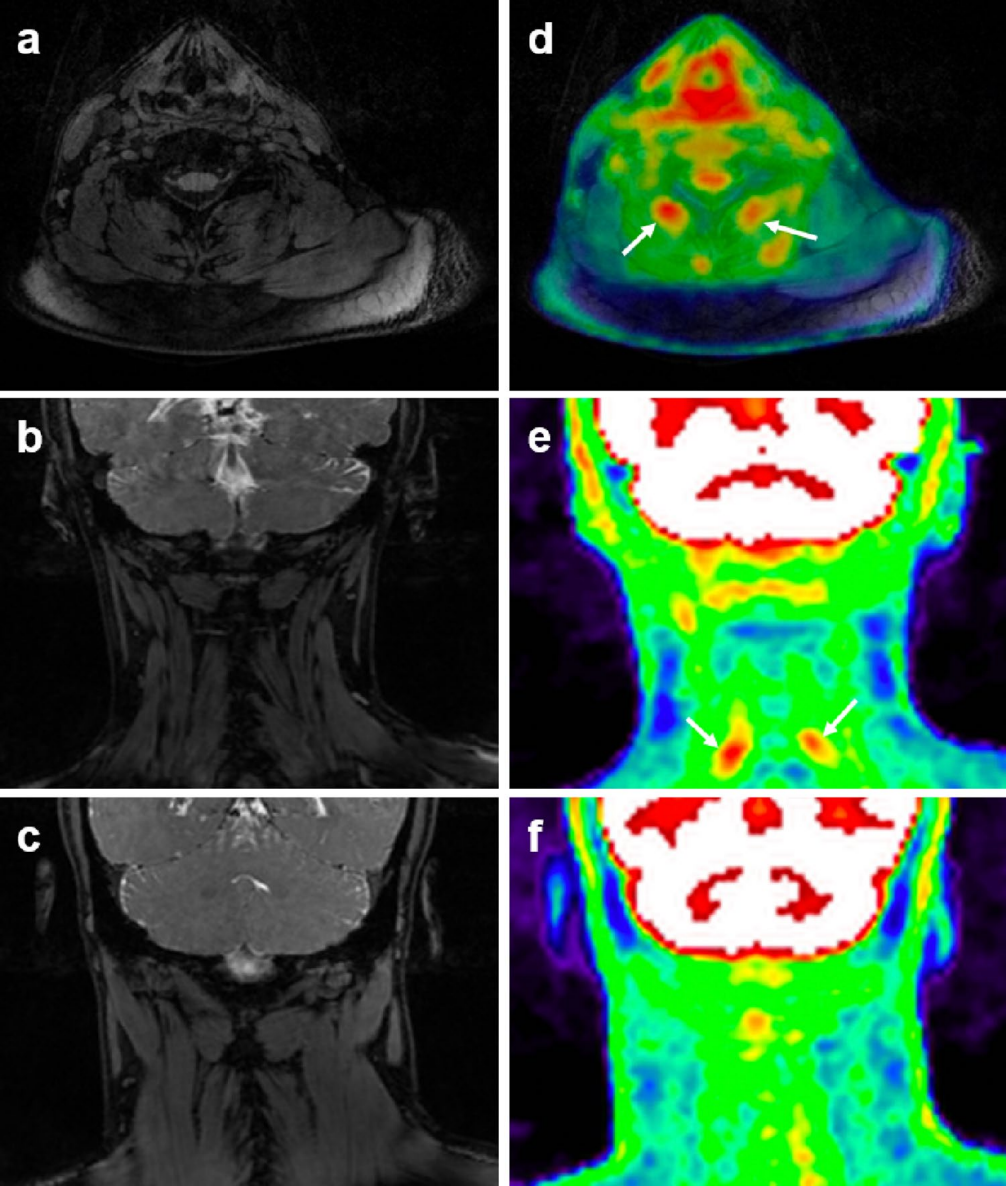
Increased [18F]FDG uptake in cervical paraspinal muscles of patient with suspected cerebrospinal fluid leak. Axial MRI at C6 (a) appears normal, while the corresponding axial PET/MRI (d) shows increased [18F]FDG uptake in paraspinal muscles (arrows, mean SUV_max_ = 2.24) of a CSF-leak patient presenting with head pain. The same pattern is also shown in coronal MRI (b) and PET/MRI (e). The corresponding coronal MRI and PET/MRI images from a healthy control subject is displayed in (c) and (f), respectively. No abnormal focal uptake of [18F]FDG as high as those in the patient case is observed in the asymptomatic subject (mean SUV_max_ = 1.38).
